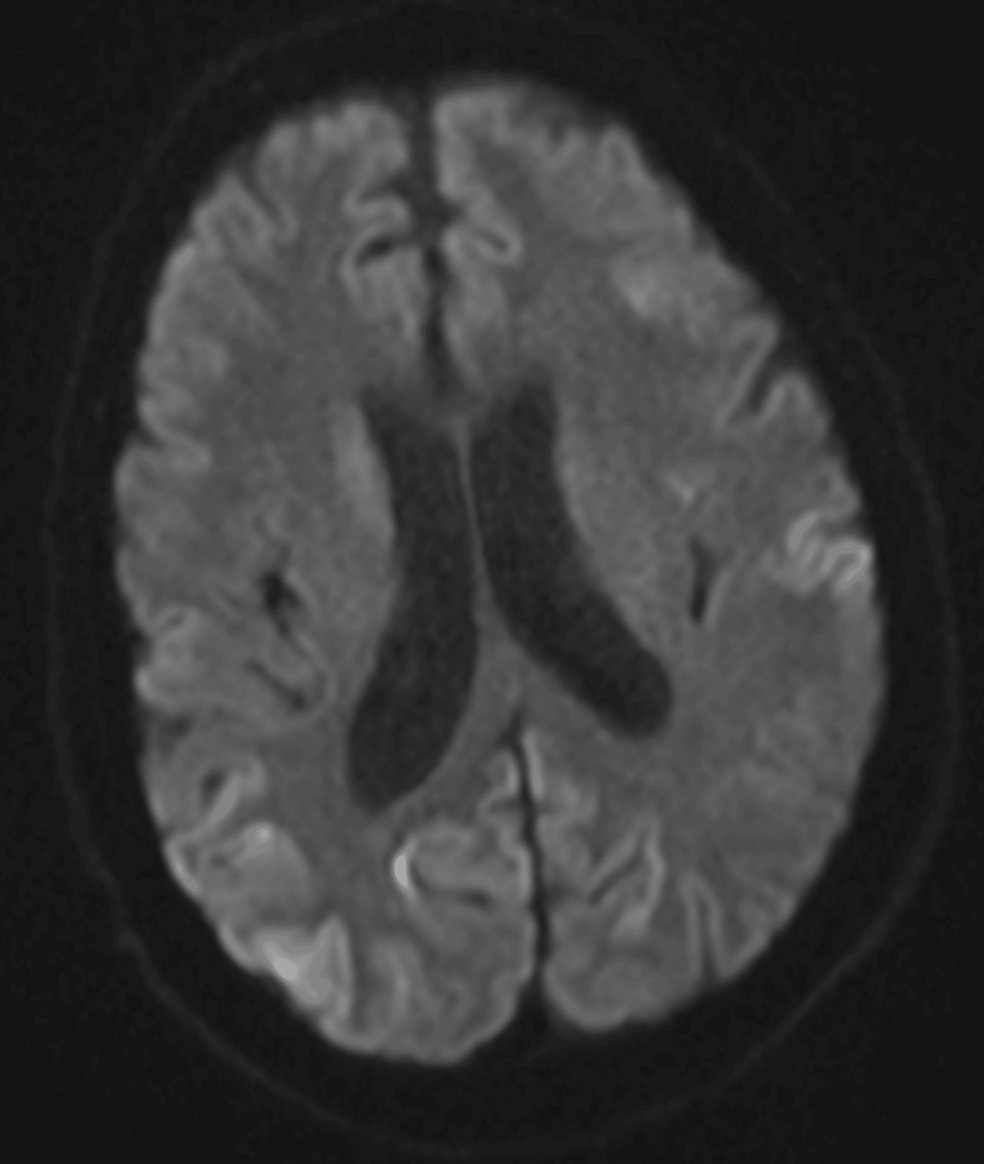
MRI showing diffusion restriction in bilateral hemispheres on DWI DWI: diffusion-weighted Imaging
